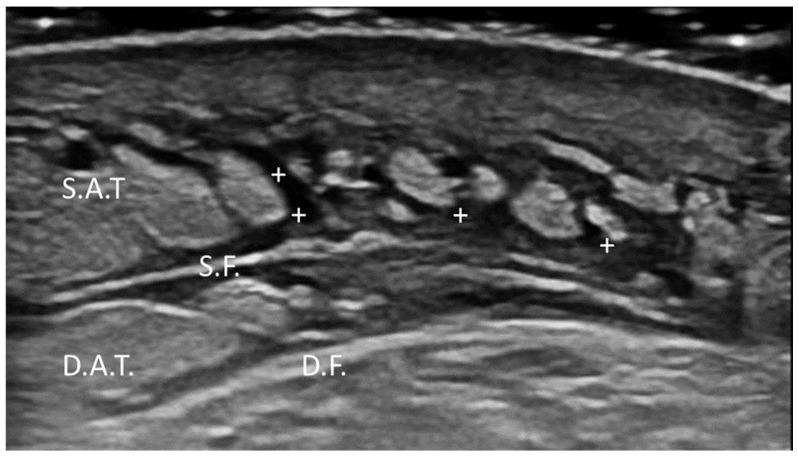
Ultrasonographic features of lymphedema in the anterior arm. US image demonstrates increased subcutaneous tissue thickness with characteristic honeycomb pattern, indicative of fluid accumulation between the retinacula cutis. +: fluid accumulation. S.F.: superficial fascia. D.F.: deep fascia. S.A.T.: superficial adipose tissue. D.A.T.: deep adipose tissue.
